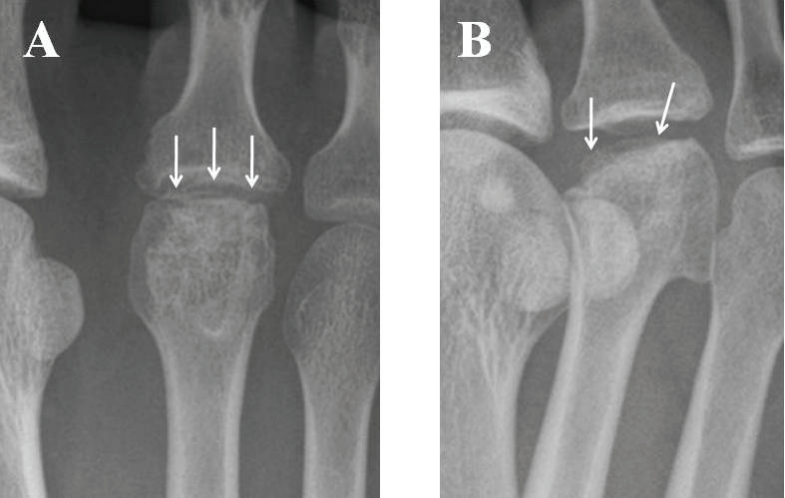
Radiographic images of the patient's metatarsophalangeal (MTP) joint two years after her operation. (A) Anteroposterior and (B) oblique views of the MTP joint of the right second toe show that the transplanted osteochondral graft was united well with the host bone of the second metatarsal head and that the articular surface of the graft had remodeled well.
